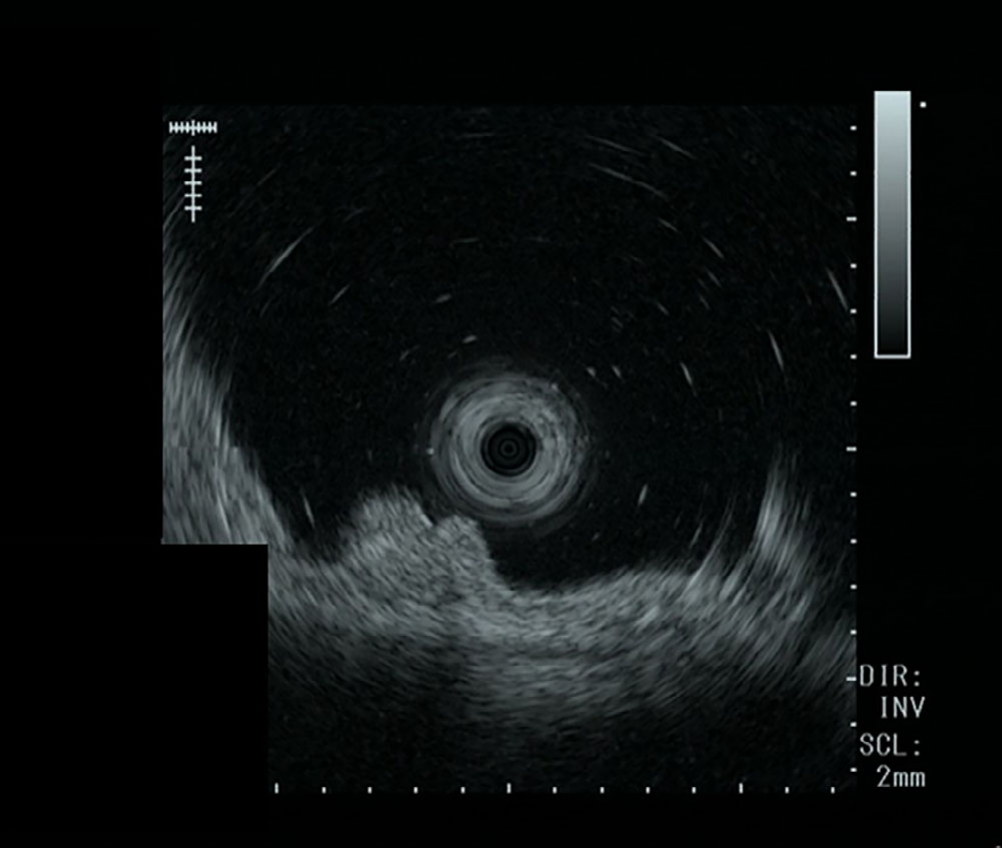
Endoscopic ultrasonography (EUS) image. EUS revealed a 5‐mm hypoechoic tumor within the submucosal layer.
